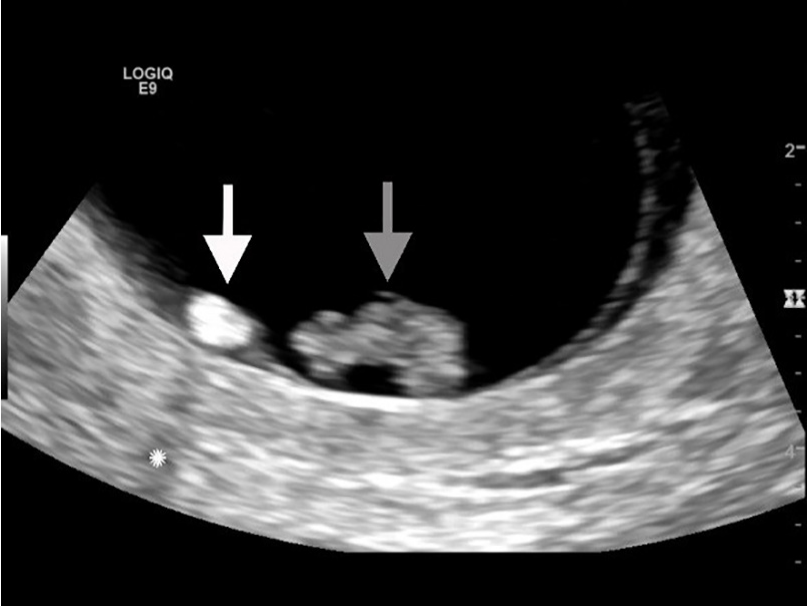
Close-up view of transvaginal sonogram demonstrating a fetal pole (gray arrow) with an adjacent echogenic yolk sac (white arrow), along with posterior acoustic shadowing (asterisk).
